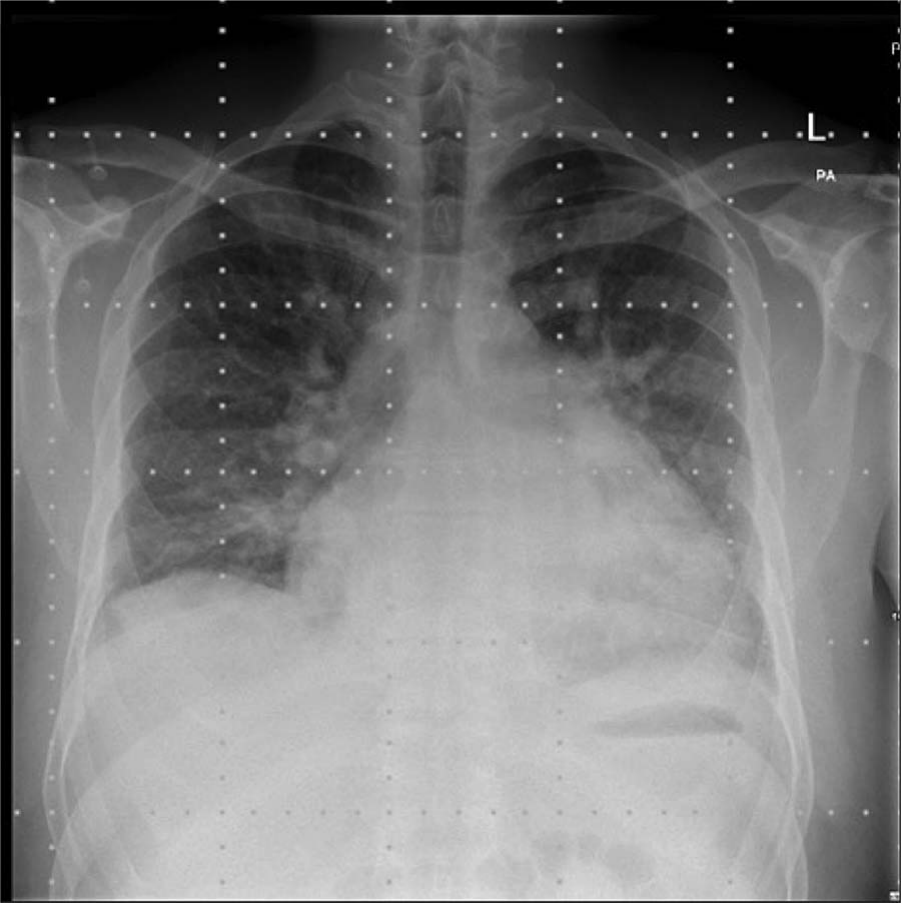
Pre-operative chest radiograph.
